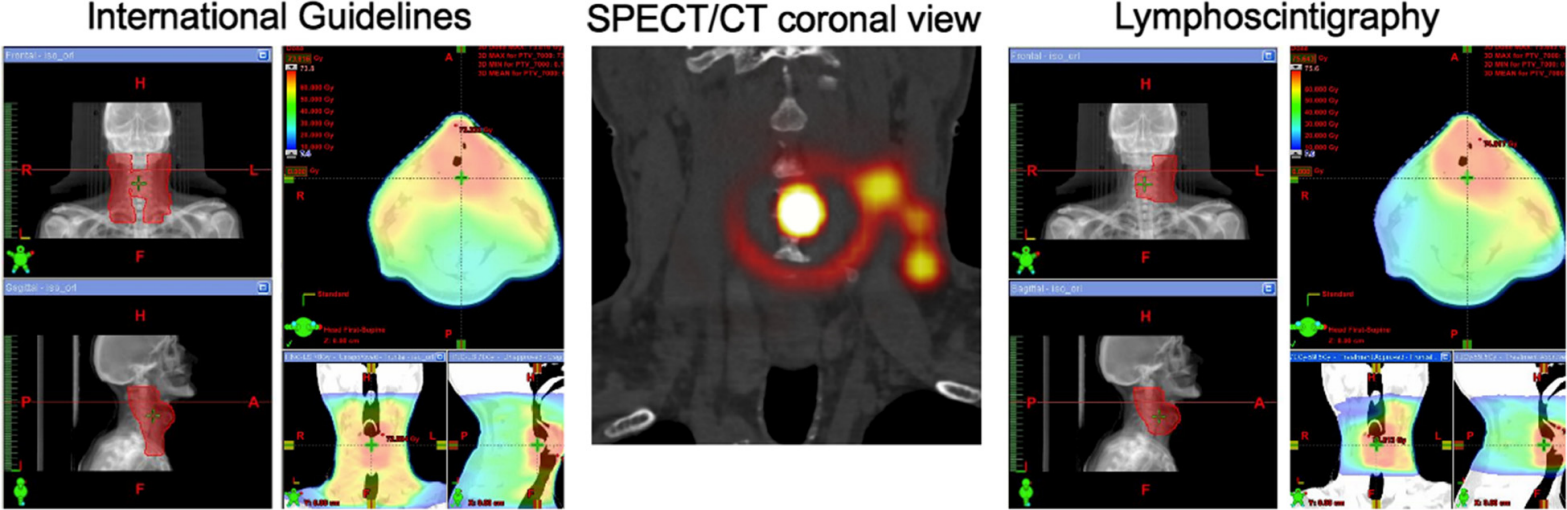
Impact of lymphoscintigraphy on volumes selection and dose distribution for patient 2. Left: PTV based on international guidelines projected on Digitally Reconstructed Radiographies (DRR) and correlated 3D dose distribution. Centre: SPECT/CT coronal view depicting 1 node caudally in level 2 left and 2 other ones in level 3 left. Right: PTV selection based on lymphoscintigraphy projected on DRR and correlated 3D dose distribution.
